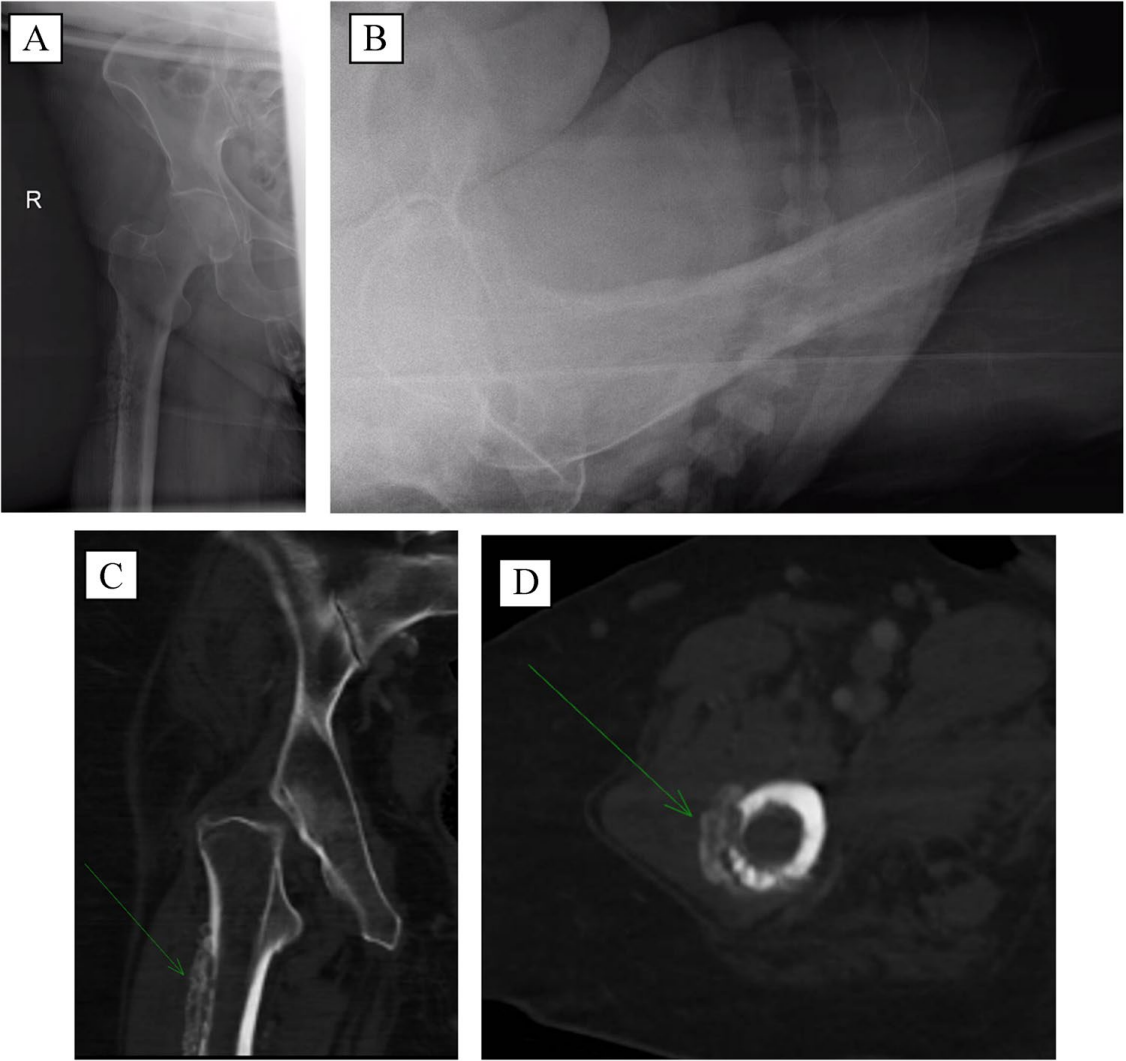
4/2021 — AP (A) and lateral (B) right hip radiographs demonstrating an infiltrative lesion involving the right proximal femoral diaphysis with complete lateral cortical destruction and adjacent periosteal reaction consistent with impending pathologic fracture. Coronal computed tomography (CT) (C) and axial CT (D) similarly showing lateral proximal diaphyseal cortical destruction with adjacent exuberant lateral cortical periosteal reaction and osseous proliferation (see arrows)
